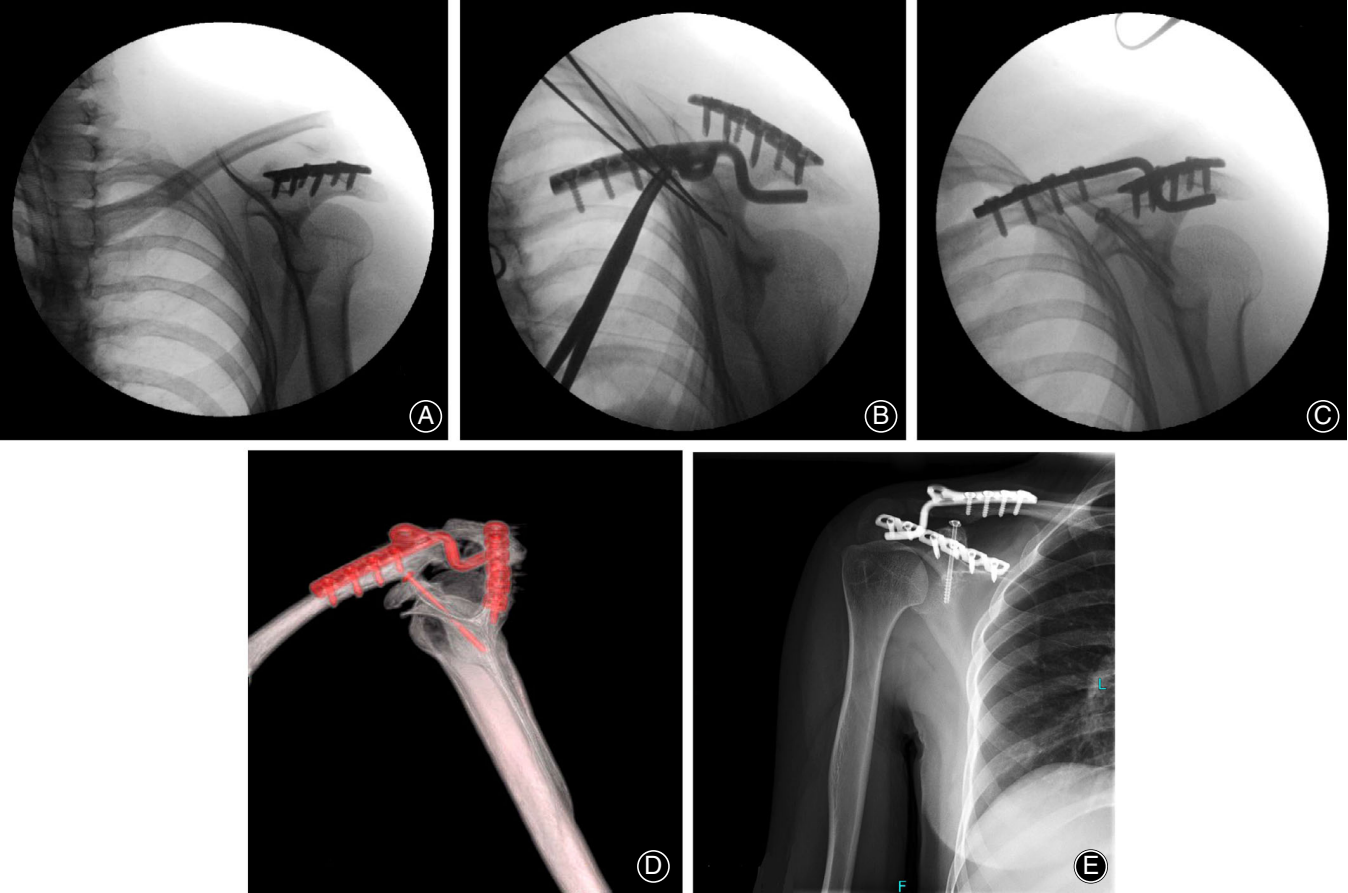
(A) Reduction and fixation of the acromion fracture with a 3.5 mm reconstruction locking plate; (B) placement of the clavicular hook plate; (C) insertion of the cannulated lag screw along the guide wire; (D) postoperative three‐dimensional CT showing the anatomic reconstruction of the disrupted superior shoulder suspensory complex (SSSC); (E) anatomical fracture healing and intact hardware 2 years postsurgery; there is erosion under the acromion due to the hook plate.
